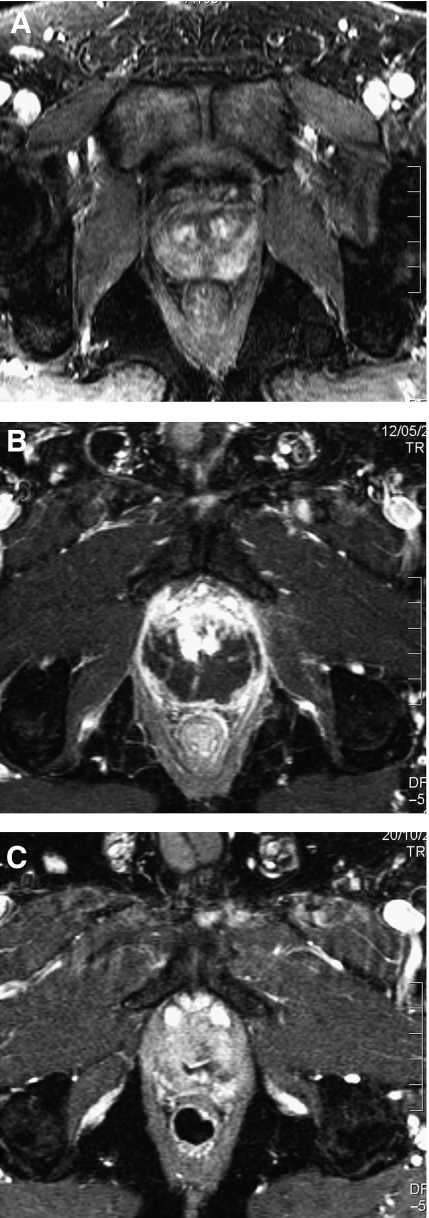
Contrast enhanced MRI changes in an under-treated prostate following HIFU. (A) 1.5 Tesla dynamic contrast enhanced MRI using gadolinium prior to HIFU treatment demonstrating localised disease with a lesion in the left postero-lateral side of the gland (circled). (B) 1.5 Tesla dynamic contrast enhanced MRI using gadolinium at 2 weeks demonstrating poor perfusion in the posterior prostate after HIFU treatment but an area of residual tissue with enhancement anteriorly (circled). (C) 1.5 Tesla dynamic contrast enhanced MRI using gadolinium at 6 months showing residual prostate tissue (circled).
